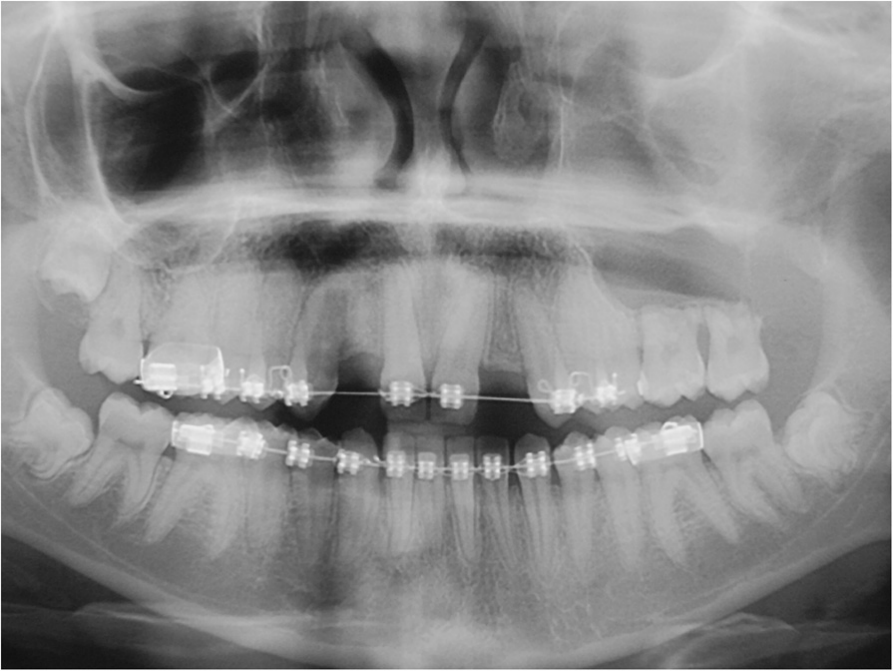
Dental panoramic radiograph.
